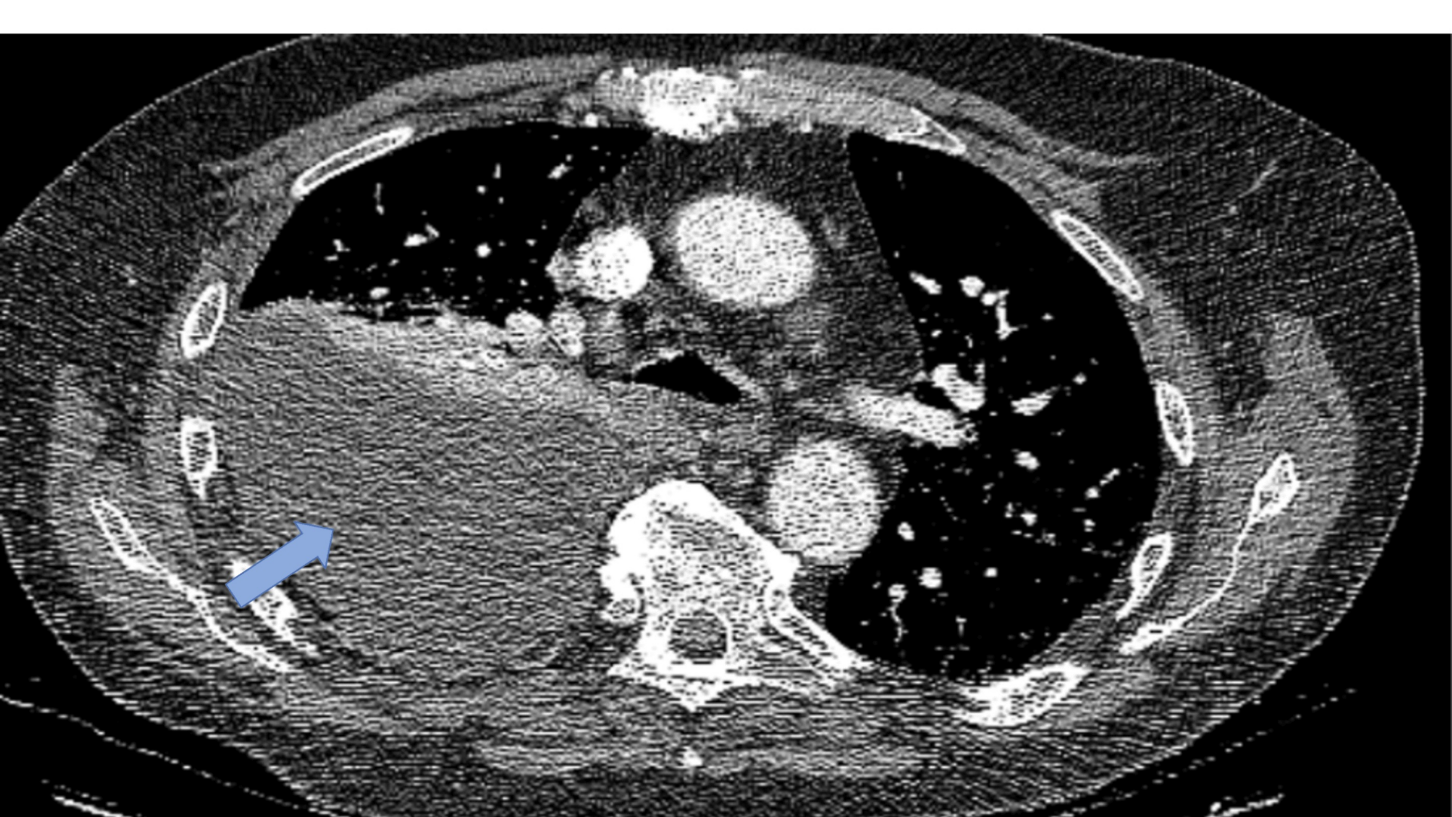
CT scan of the chest (axial plane, 25th March, 2025). The scan demonstrates a marked increase in the size of the right pleural effusion (blue arrow) compared with the scan performed four days earlier. There is a progressive and complete collapse of the right upper and middle lobes. Central fluid-filled cystic areas within the right lower lobe are less demonstrated due to surrounding consolidation/collapse.
